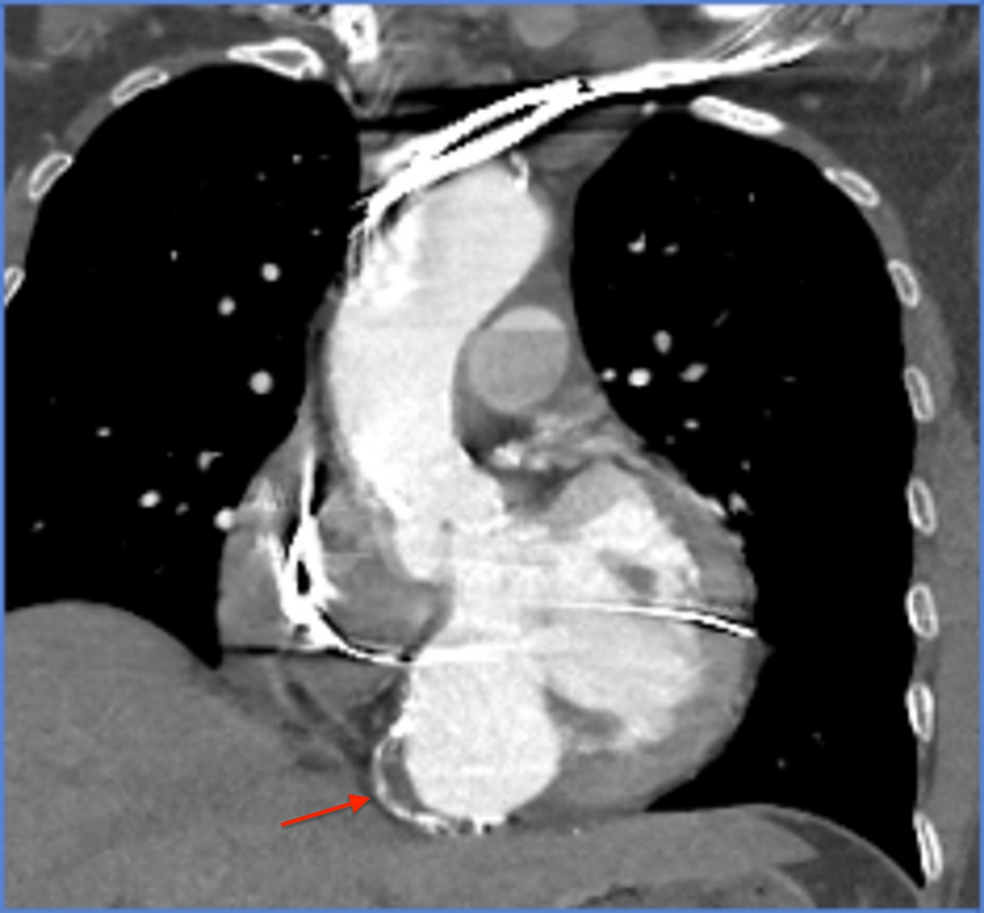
Cardiac computed tomography with angiography of the chest showing a large left true ventricular aneurysm.
